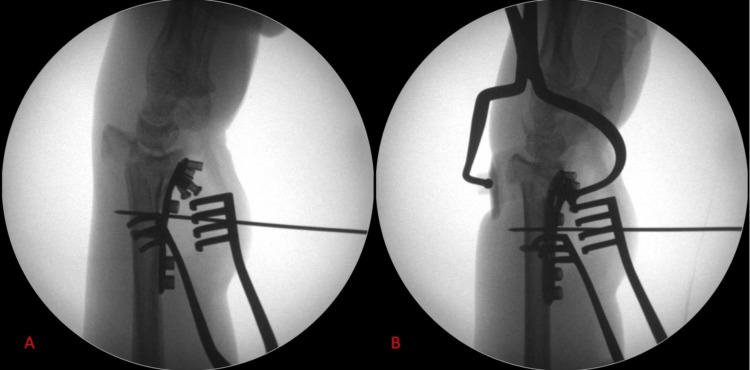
Example of the use of the soft tissue bone reduction clamp (A) Intraoperative lateral radiograph showing displacement of dorsal fragment (B) After application of the reduction clamp, there is near-complete reduction of the dorsal fragment.
